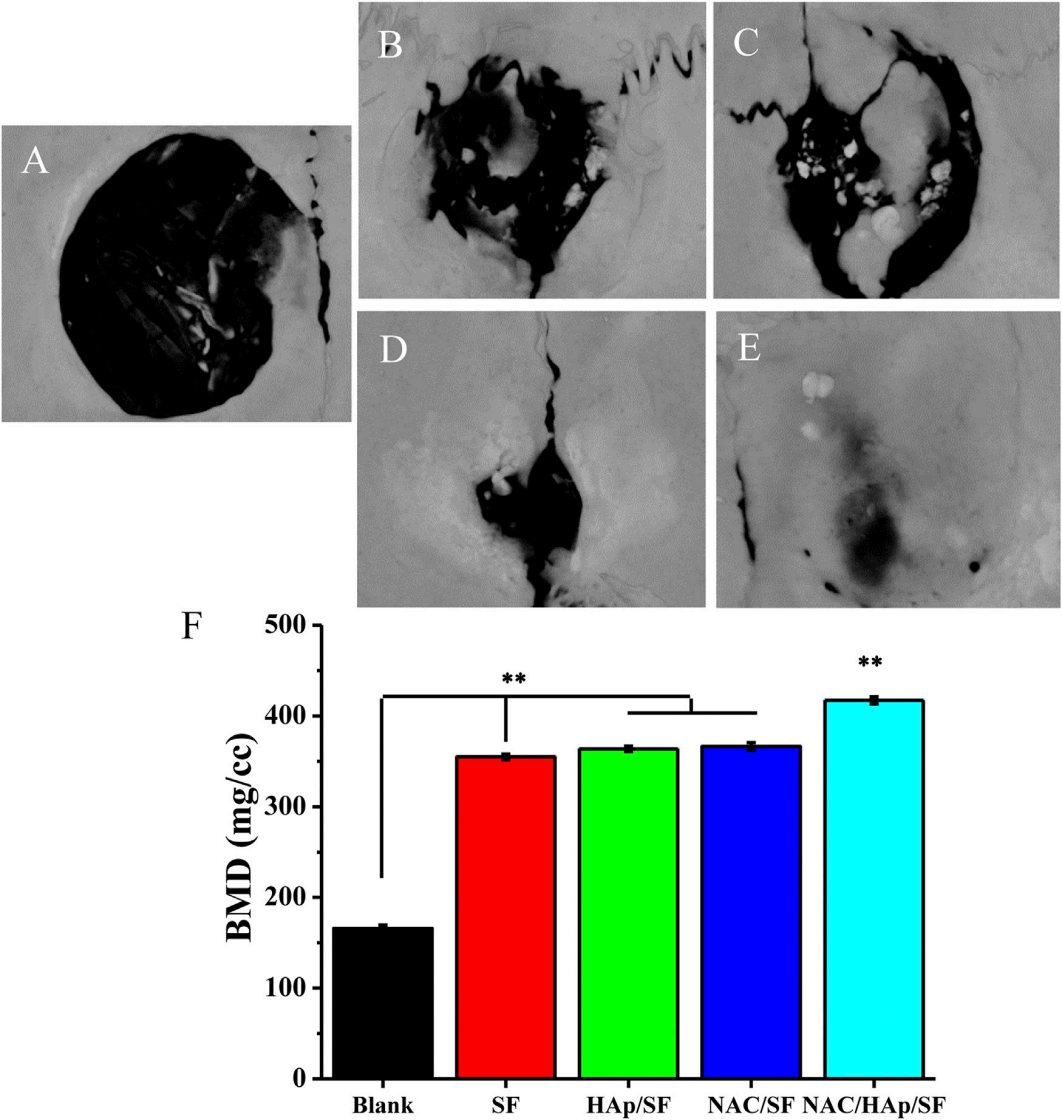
CT examination of the whole calvarias (A–E) and bone mineral density of the defects (F) 8 weeks after implantation *in vivo*
